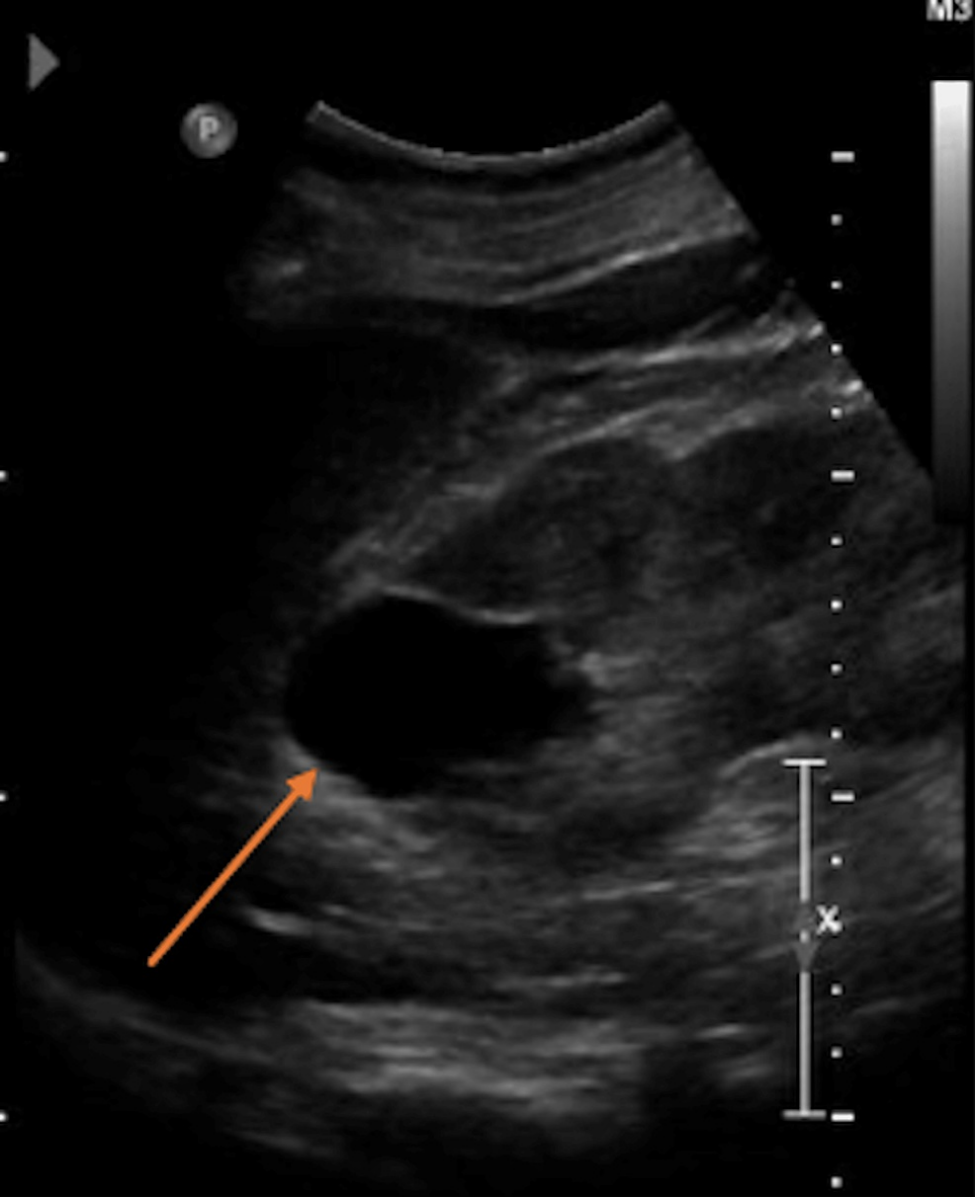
Renal ultrasound Renal ultrasound showed a 4.8 x 3.0 x 3.0 cm thin-walled cyst in the upper pole of the left kidney (orange arrow).
